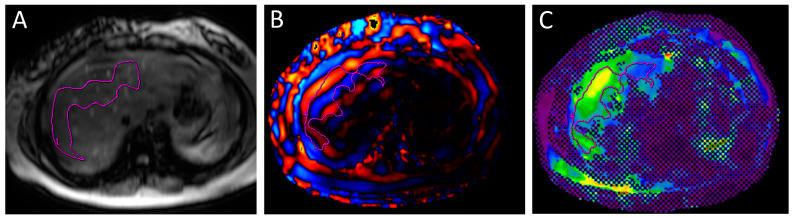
Images from an MRI elastography image showing the (A) magnitude images; (B) wave images; and (C) elastography map. A region of interest (ROI) was manually drawn on the elastogram image over the right lobe of the liver (purple), taking care to avoid the hexed-out areas. The ROI was propagated onto the wave, and magnitude image and adjustments were made to avoid large vessels.
